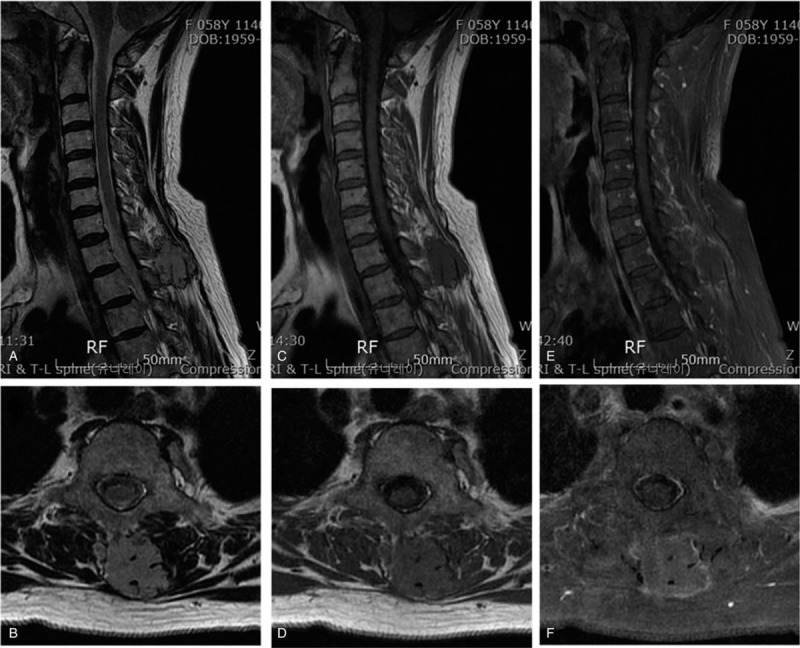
Preoperative magnetic resonance imagings demonstrated a round shaped, multi-lobulated, and well-defined mass lesion embedded from the inter-spinous space at the T1-2 level to the left paraspinal muscles. The lesion was iso-intense on T2-weighted images (WI) (A, B), iso- to slightly low-intense on T1-WI (C, D), heterogeneous enhancement of intra- and peri-mass lesion on contrast-enhanced T1-WI (E, F). Vascular structures presented as signal voids were identified internally and around the mass lesion.
